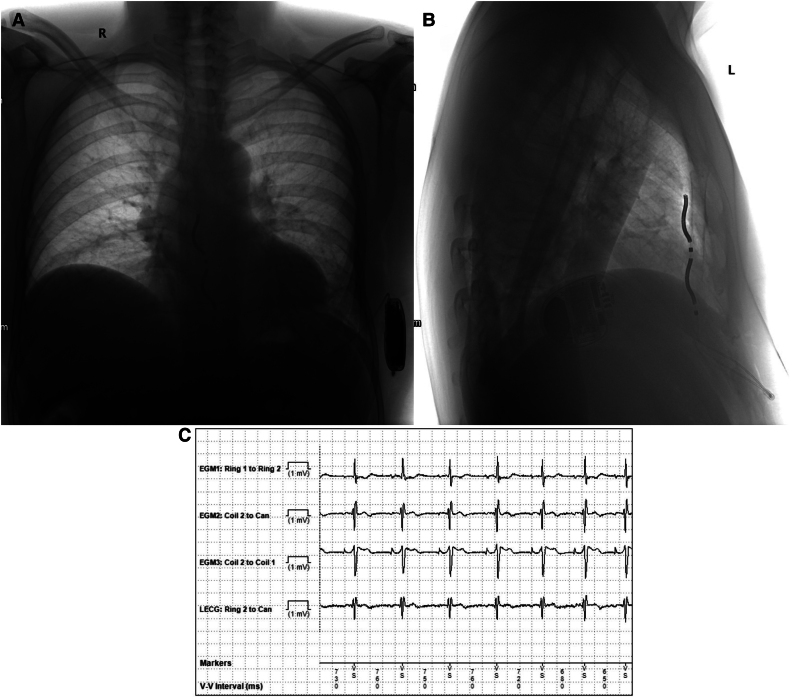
Post-procedure chest X-ray and extravascular implantable cardioverter-defibrillator electrogram—(*A*) antero-posterior chest X-ray after extravascular implantable cardioverter-defibrillator implantation. (*B*) Lateral chest X-ray highlighting position of extravascular implantable cardioverter-defibrillator lead in relation to sternum. (*C*) Extravascular implantable cardioverter-defibrillator intra-cardiac electrogram: R wave amplitude was 2.0 mV and a small p wave was visible but not detected by the device.
